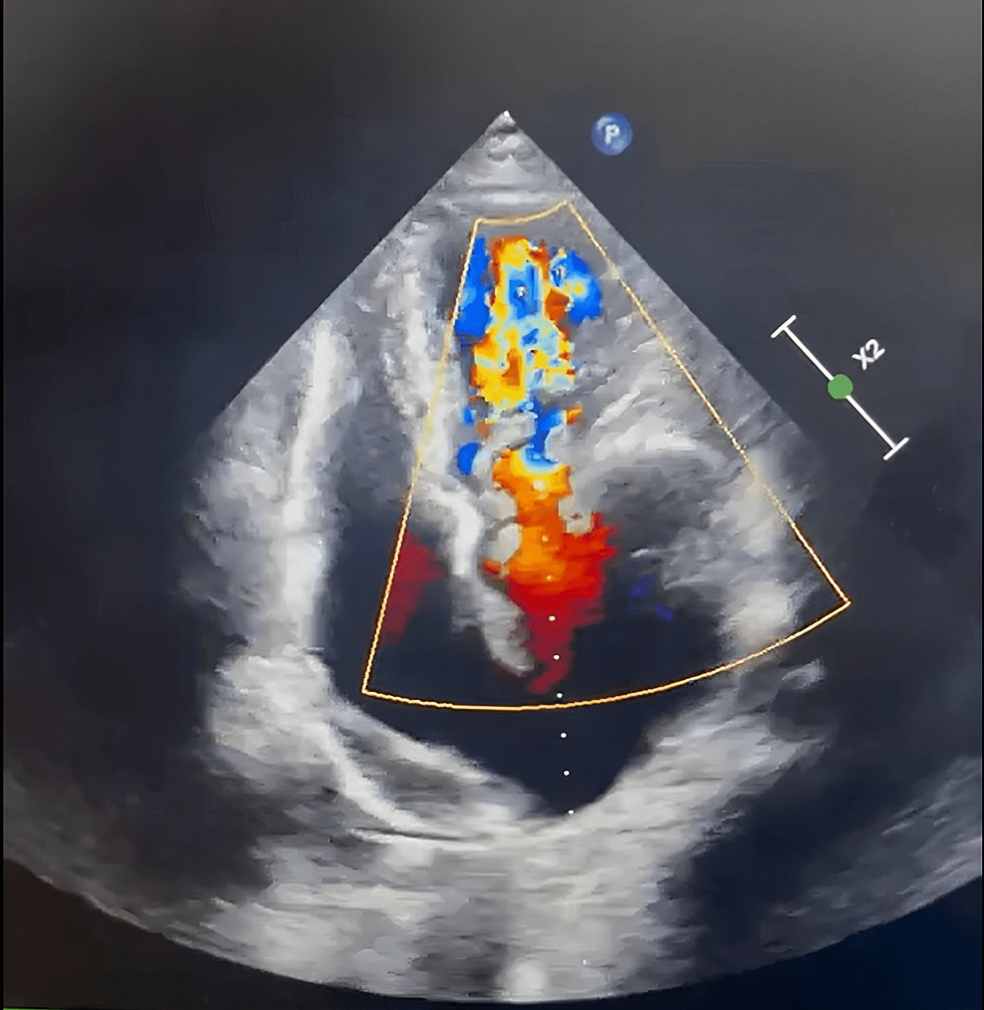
Echocardiography showing mitral stenosis and mitral regurgitation
